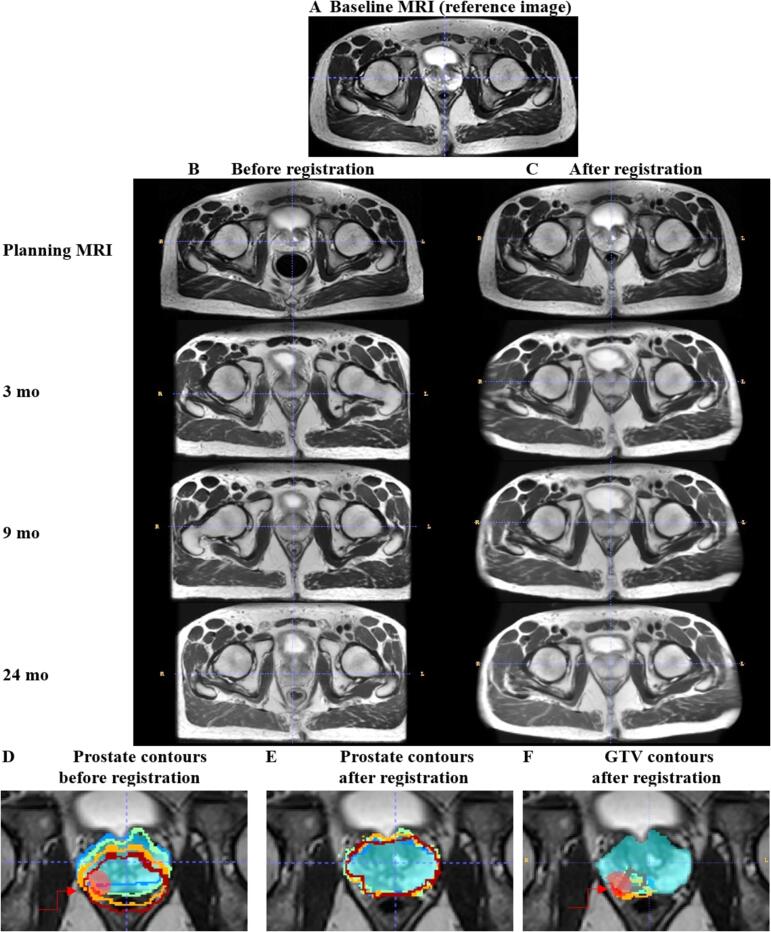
Registration of all timepoints to baseline T2w image (A). Rows 2–5: T2w images during planning MRI and post-RT before registration (B) and after registration (C) in baseline T2w space (A). All results before deformable registration shown in this figure have been produced using linear alignment (rotation and translation). Bottom row (D-F): The prostate (cyan volume) and GTV (red volume and red arrow) as outlined in baseline T2w are superimposed with corresponding prostate and GTV contours from planning MRI (blue), 3 mo (green), 9 mo (orange), 24 mo (red) post-RT before registration (D) and after deformable registration for prostate (E) and GTV (F). (For interpretation of the references to colour in this figure legend, the reader is referred to the web version of this article.)
